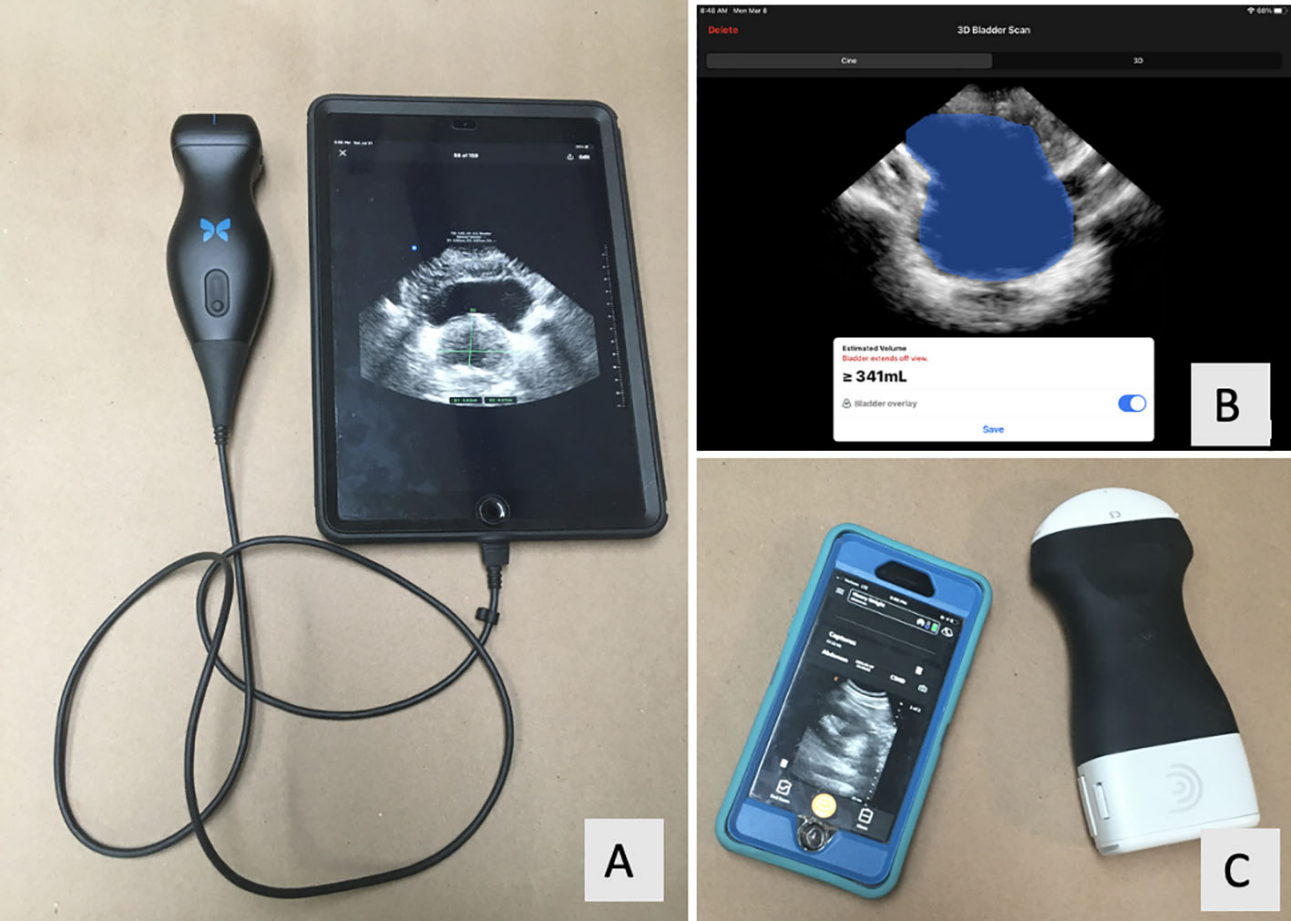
(A) Butterfly IQ probe (Guilford, CT) with the Apple iPad (Cupertino, CA). (B) Screenshot of the Butterfly IQ’s bladder scanning function. (C) Clarius C3 probe (Vancouver, BC) with Apple iPhone.
